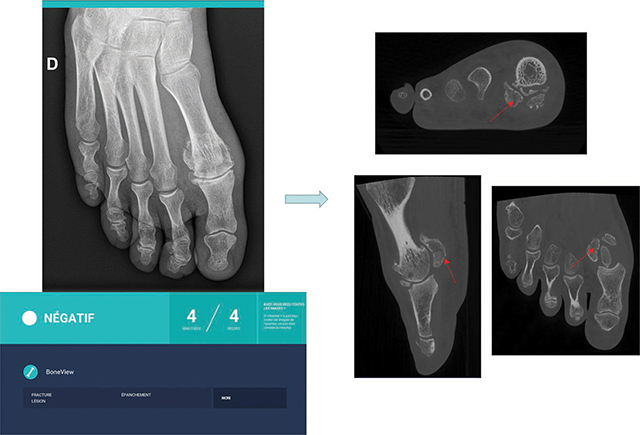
AI false negative response for a fracture of the lateral sesamoid, which CBCT confirmed.
